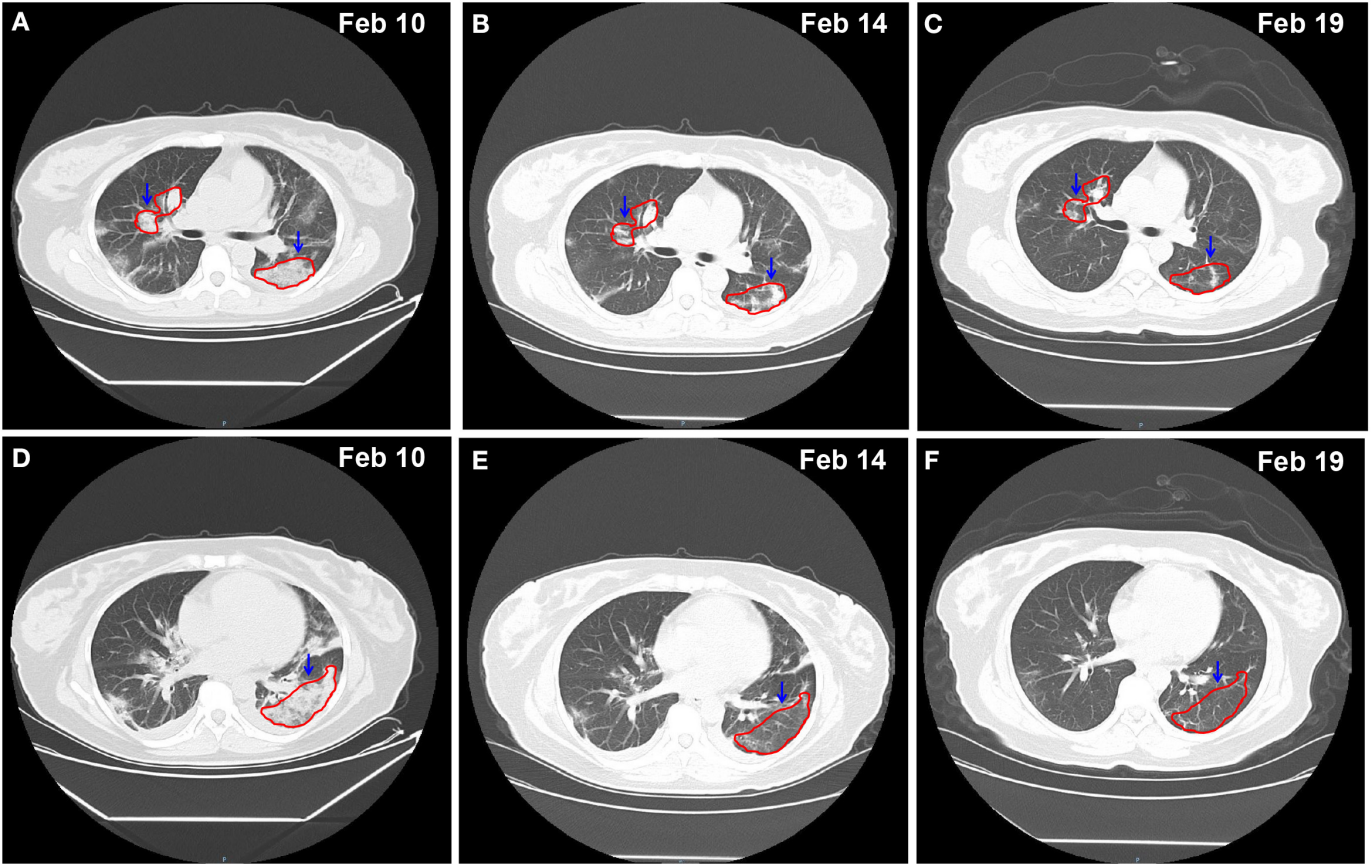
Chest CT imaging of the patient on Feb 10 -Feb 19, 2020. (A–C) pulmonary trunk as the reference coordinate, (D–F) tracheal bifurcation as the reference coordinate. CT, computed tomography. Red circle indicates the focus of lesion.
